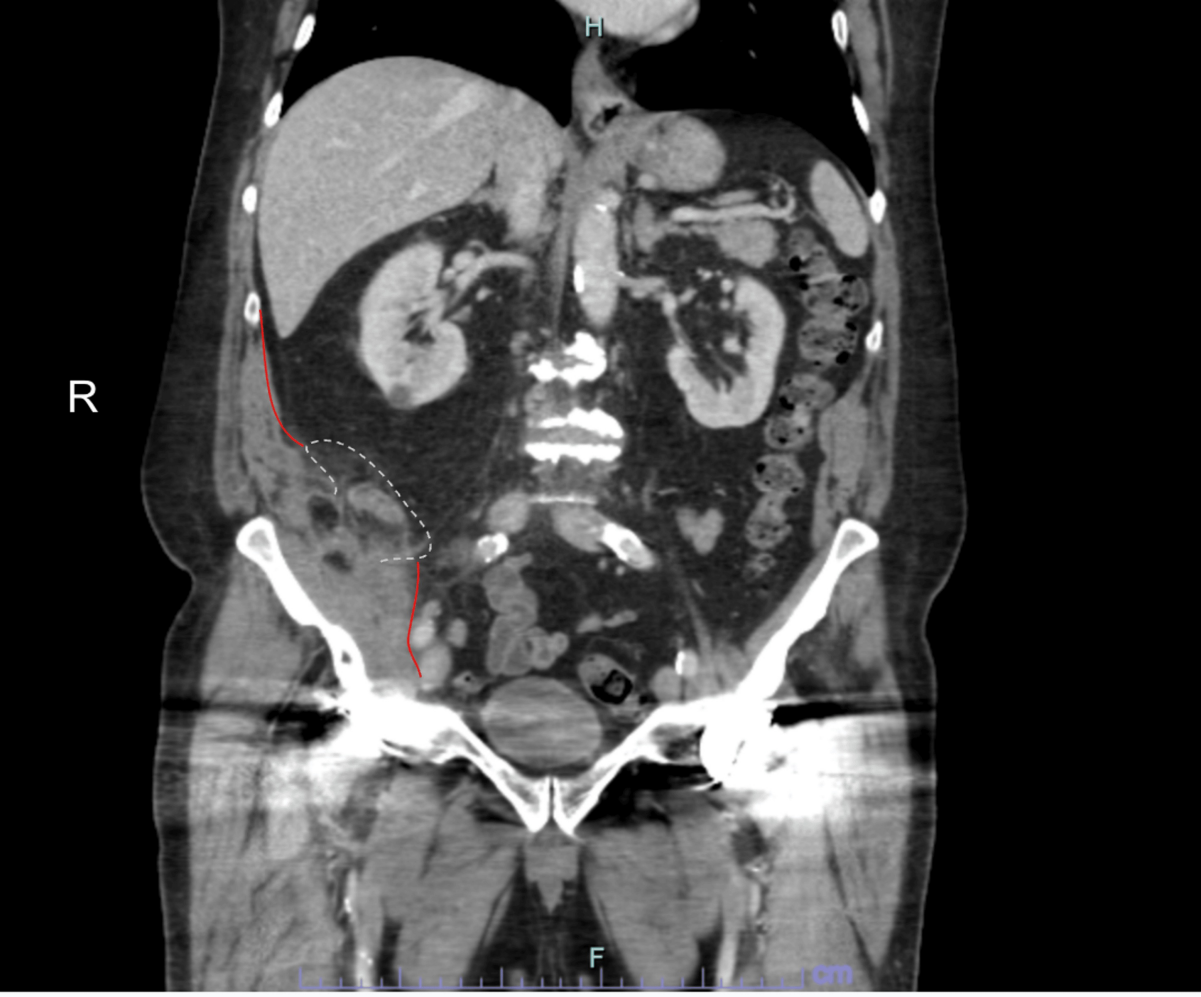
Coronal view CT depicting the appendix fistulized to the iliopsoas accompanied by abscess formation. White dotted line: border of the appendix. Red line: border of the iliopsoas.
